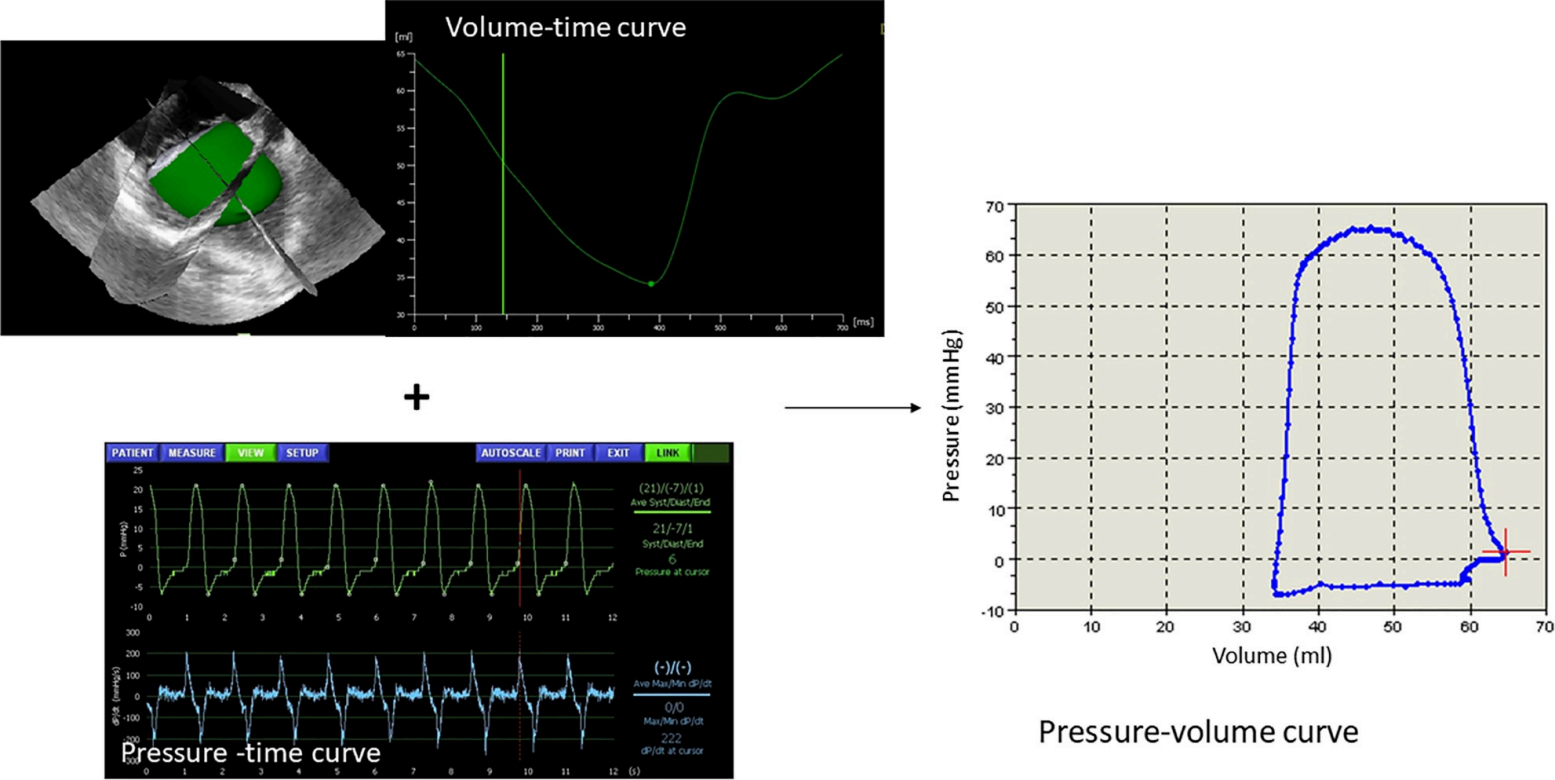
Example of a volume-time curve assessed by three-dimensional echocardiography and the ventricle cast reconstruction. In combination with the simultaneously acquired pressure-time curve a pressure-volume curve is obtained.
